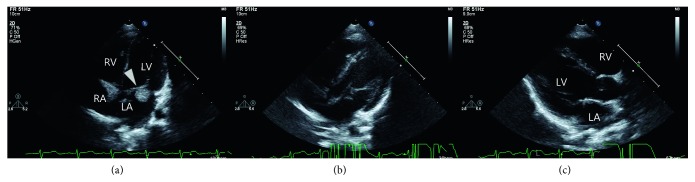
Two-dimensional follow-up echocardiography showed that rhabdomyoma size was significantly decreased at 6 months of age (a). At 3 years of age, a very small (6.5 × 2.3 mm), ellipse-shaped rhabdomyoma attached to the MV anterior leaflet without LV inlet obstruction (peak velocity = 0.76 m/sec) (b and c) was observed.
